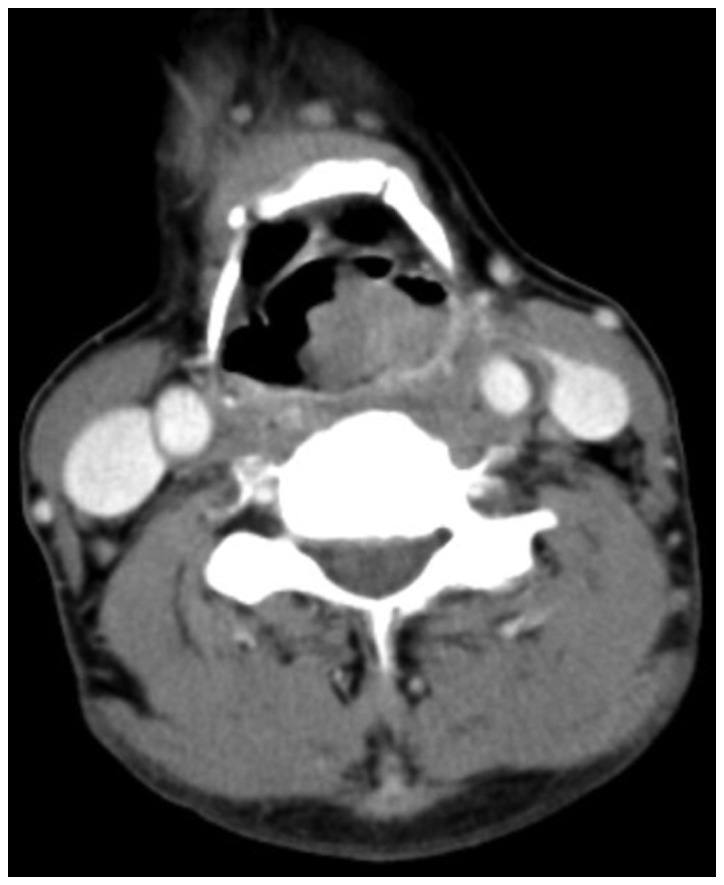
Computed tomography findings of case three showed a 3.3×4.0-cm mass on the posterior wall of the hypopharynx.
